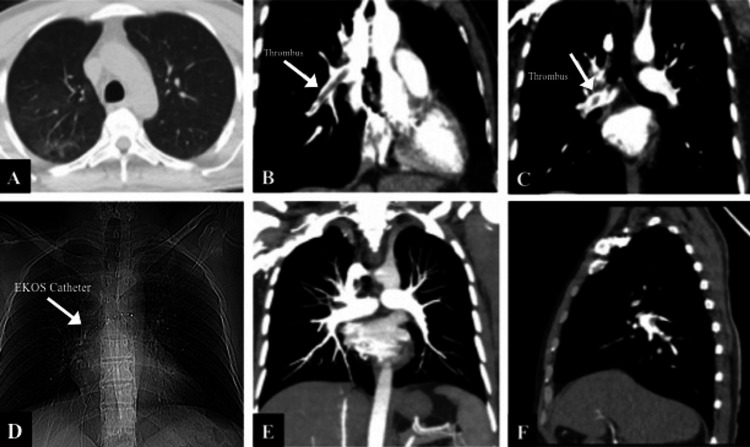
The CT findings before the EKOS treatment (A, B, C ) and at the 12th hour of the EKOS treatment (D, E, F) A: Ground-glass opacities in the right lobe; B: Massive thrombus in the right pulmonary artery; C: Filling defects due to thrombus in the segmental branches; D: View of the EKOS catheter; E: Totally cleared the right pulmonary artery and anterior truncus; F: Thrombus fragments in the posterior ascending artery and the common basal arteries EKOS: The EkoSonic™ Endovascular System (EKOS) (Boston Scientific Corporation, Marlborough, USA)
